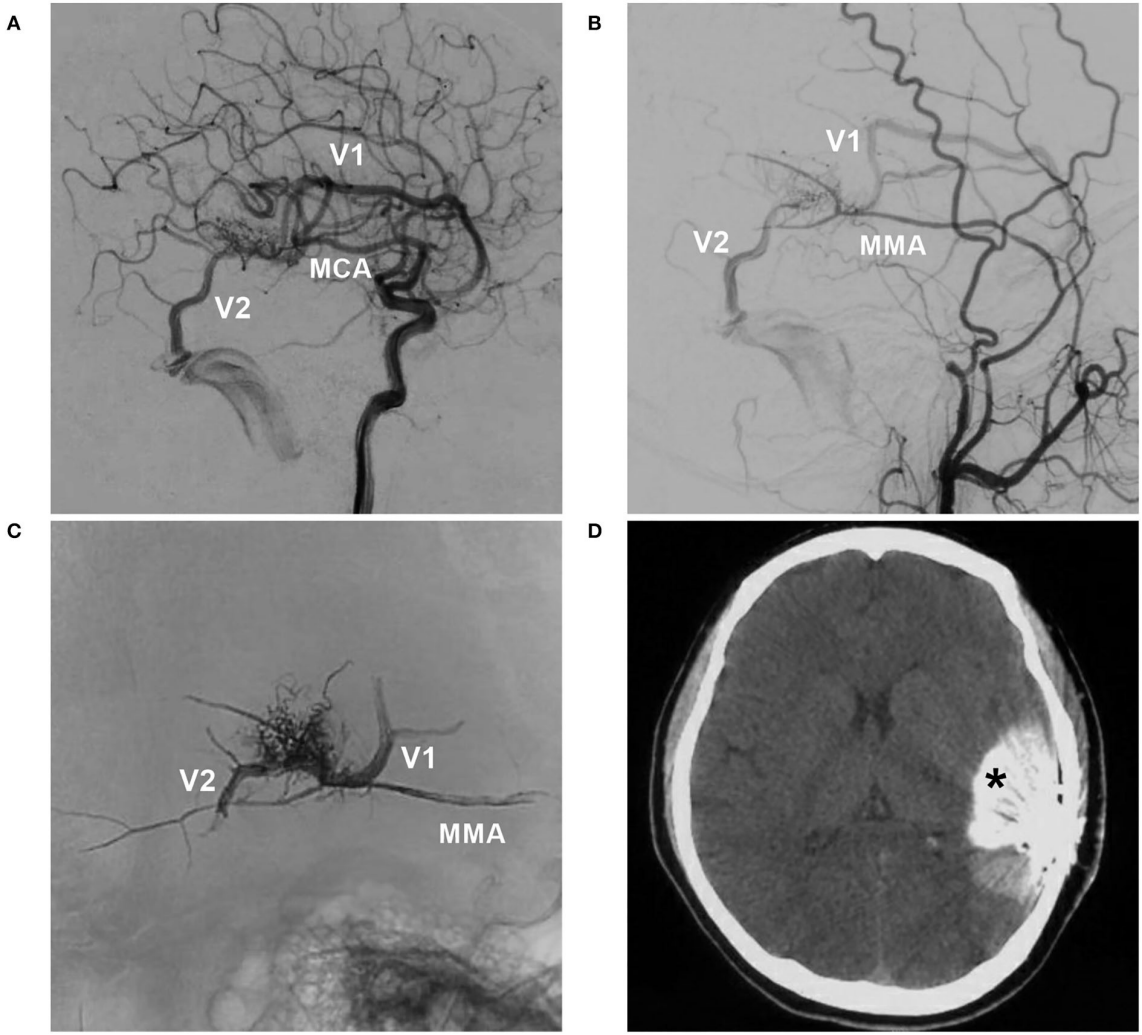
BAVM with intraoperative nidus rupture (case 18). (A,B) Arterial-phase angiograms of the internal carotid artery and external carotid artery showing the BAVM supplied by the MCA and MMA, with two draining veins (V1 and V2). (C) X-ray film showing Onyx casting *via* the MMA and occlusion of the two draining veins. (D) CT showing contrast agent extravasation (asterisk). BAVM, brain arteriovenous malformation; MCA, middle cerebral artery; MMA, middle meningeal artery.
